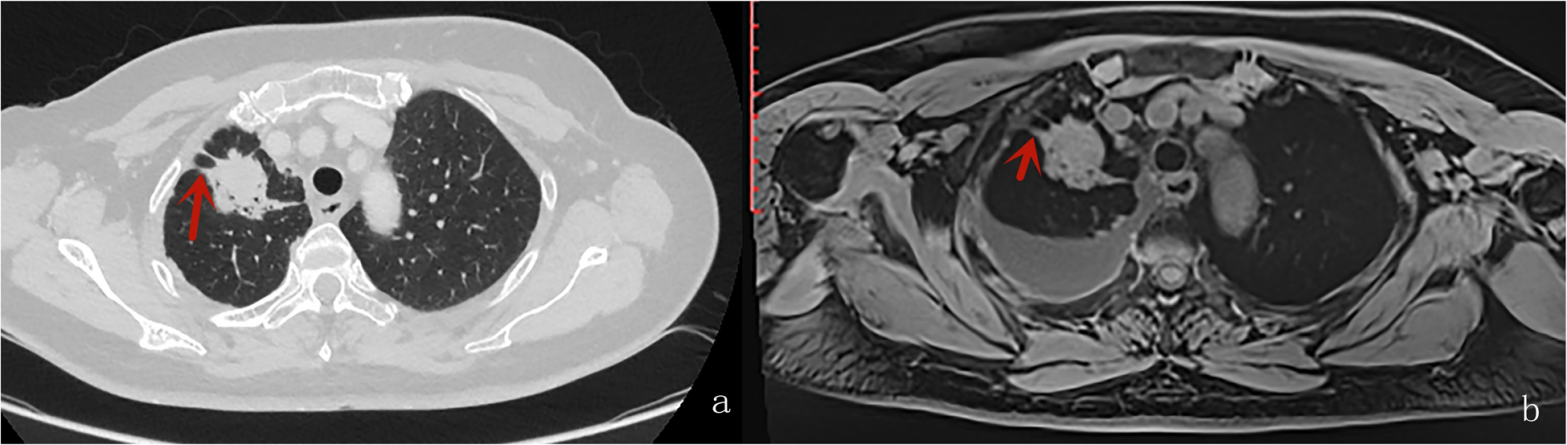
A female with adenocarcinoma; pleural indentation was found (the red arrow) in axial CT (A) and MR (B), the right pleural effusion was seen in r-VIBE obtained 1 day after CT
